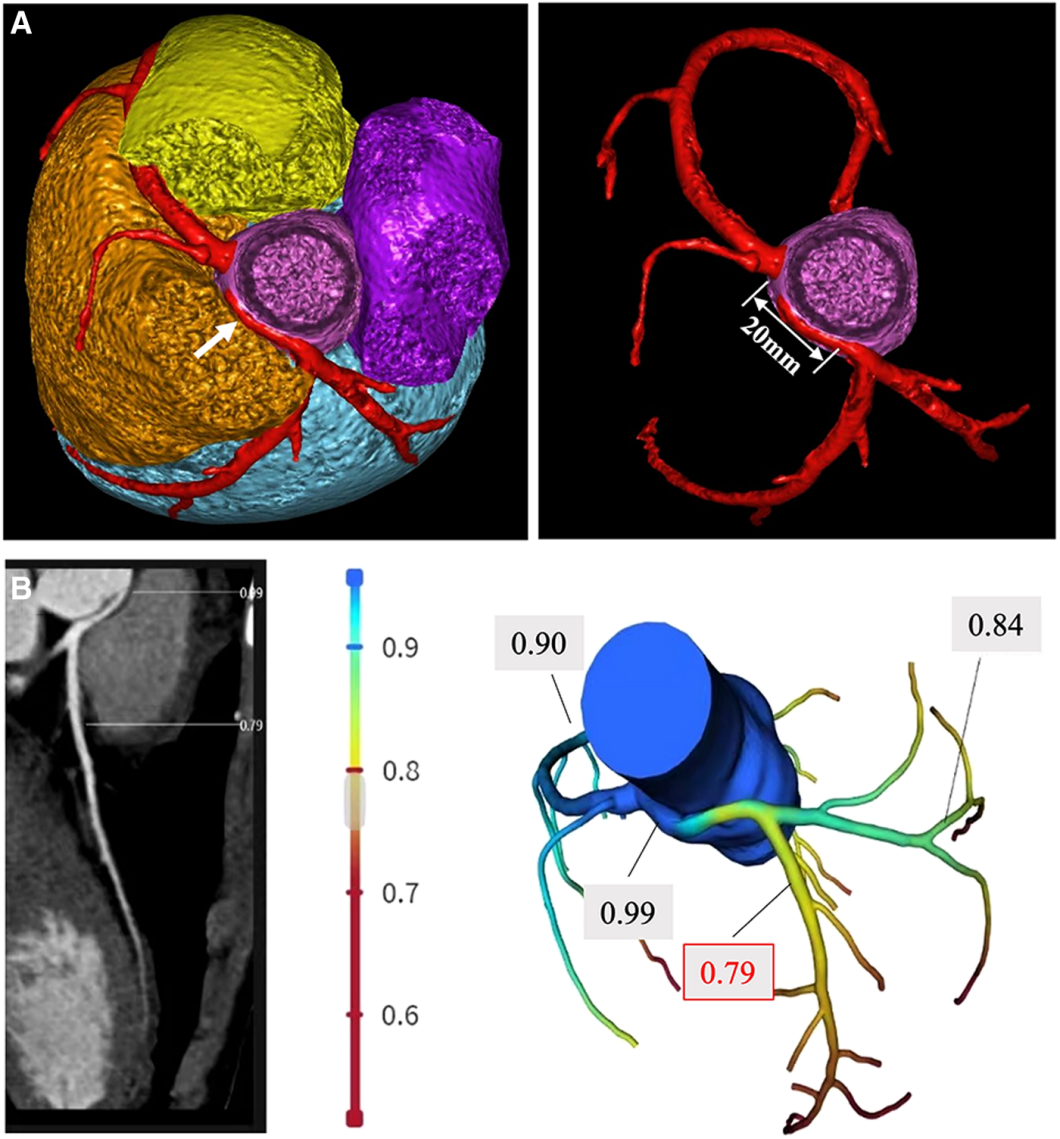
Preoperative CT-FFR measurement. (A) Coronary CTA reveals the left coronary artery originating from the right sinus of Valsalva and running between the aorta and the pulmonary artery (white arrow), the length of the intra-arterial wall course is approximately 20 mm, and the origin of the right coronary artery is normal. (B) CT-FFR measurement shows a reduced FFR of the left coronary artery (LCA, 0.79) and a normal FFR of the left circumflex artery (LCX, 0.84) and the right coronary artery (RCA, 0.90).
